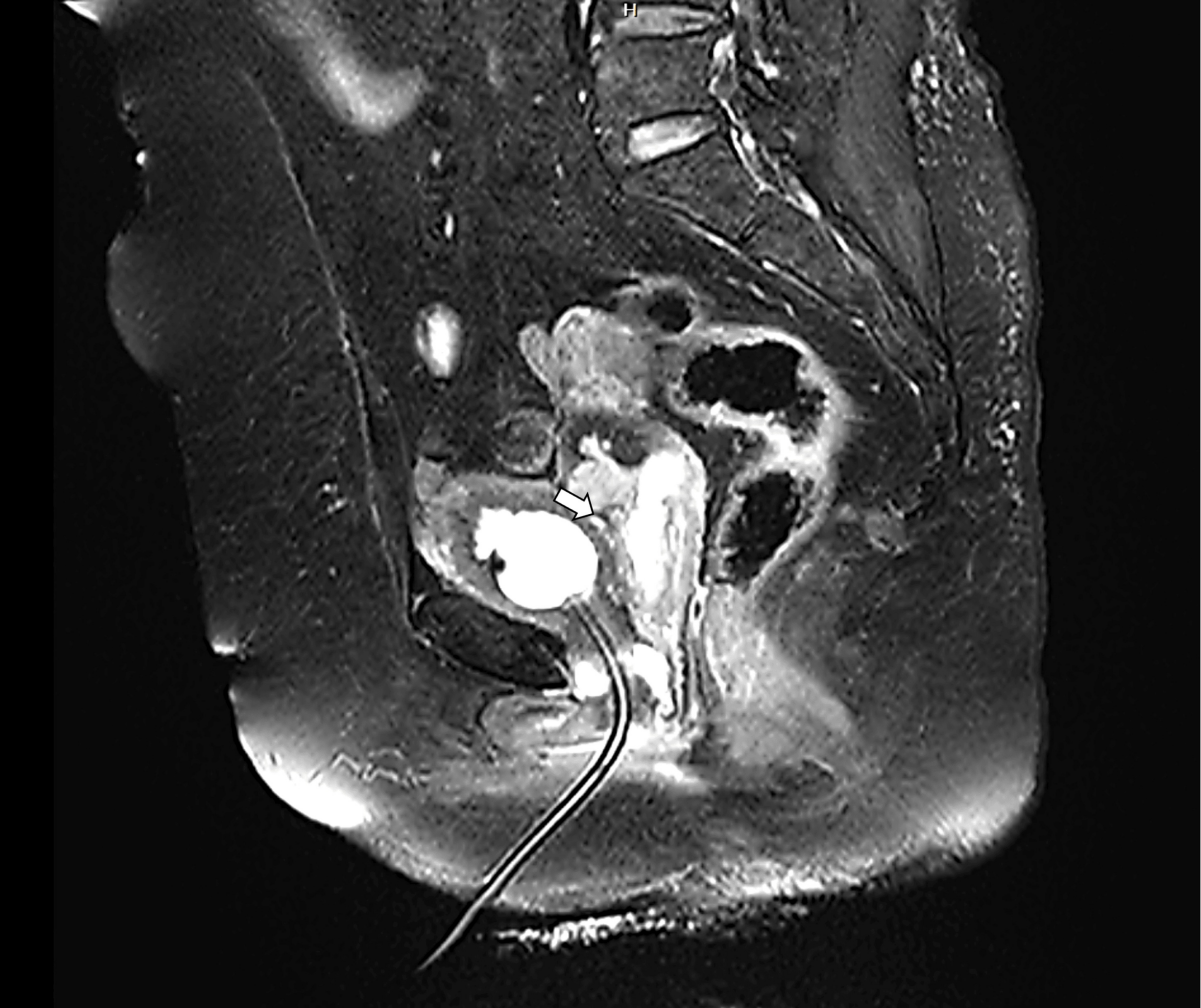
T2-weighted fast spin echo (FSE) sagittal image The arrows shows a T1 hypointense and T2 hyperintense mass localized to the anterior cervix. The uterus is postmenopausal in size with preserved surrounding stromal interfaces.
